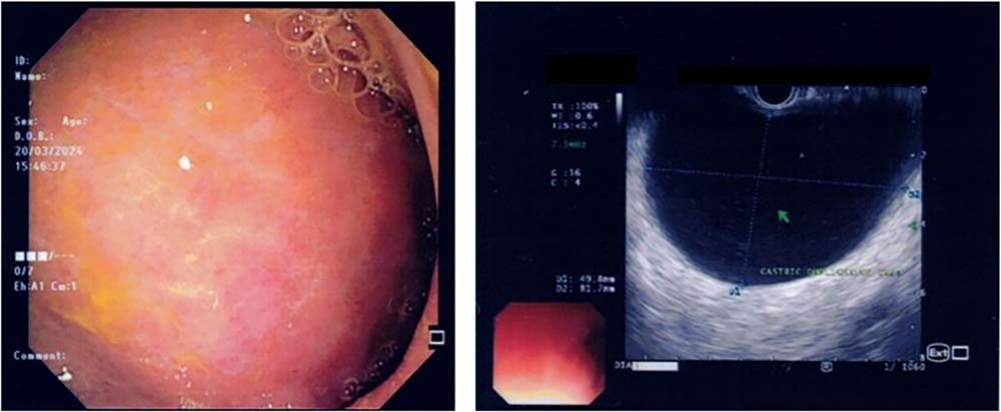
Endoscopic images demonstrate a subepithelial mass externally compressing the antrum. Endosonography revealed a well-defined, homogenous lesion with delineation across multiple gastric wall layers.
